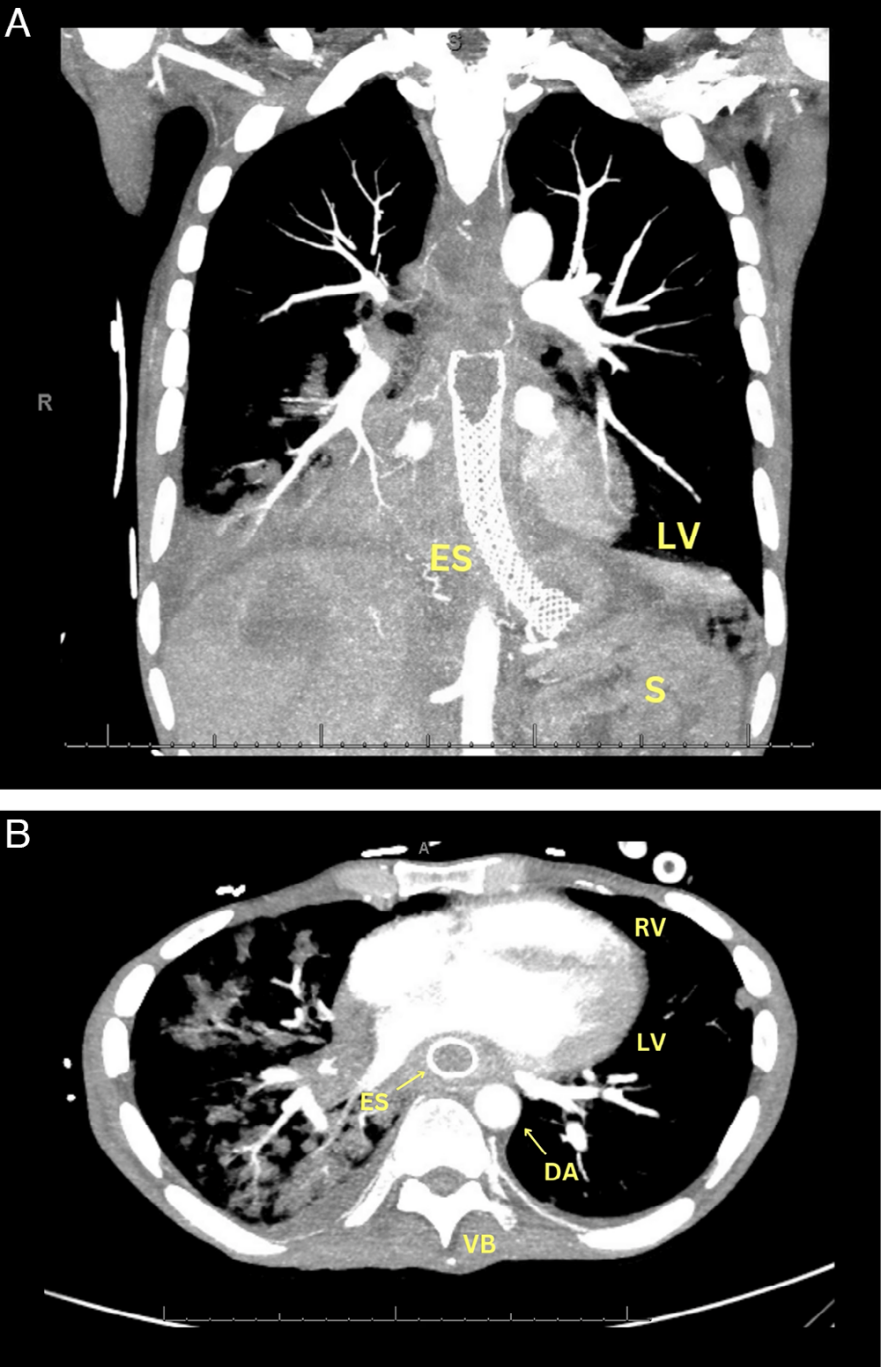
Computed tomography scans. (A) Shows the esophageal stent with the nitinol meshwork. (B) Shows the relationship of the ES to other anatomic structures. ES, esophageal stent; S, spleen. Other abbreviations are in Figure 1.
